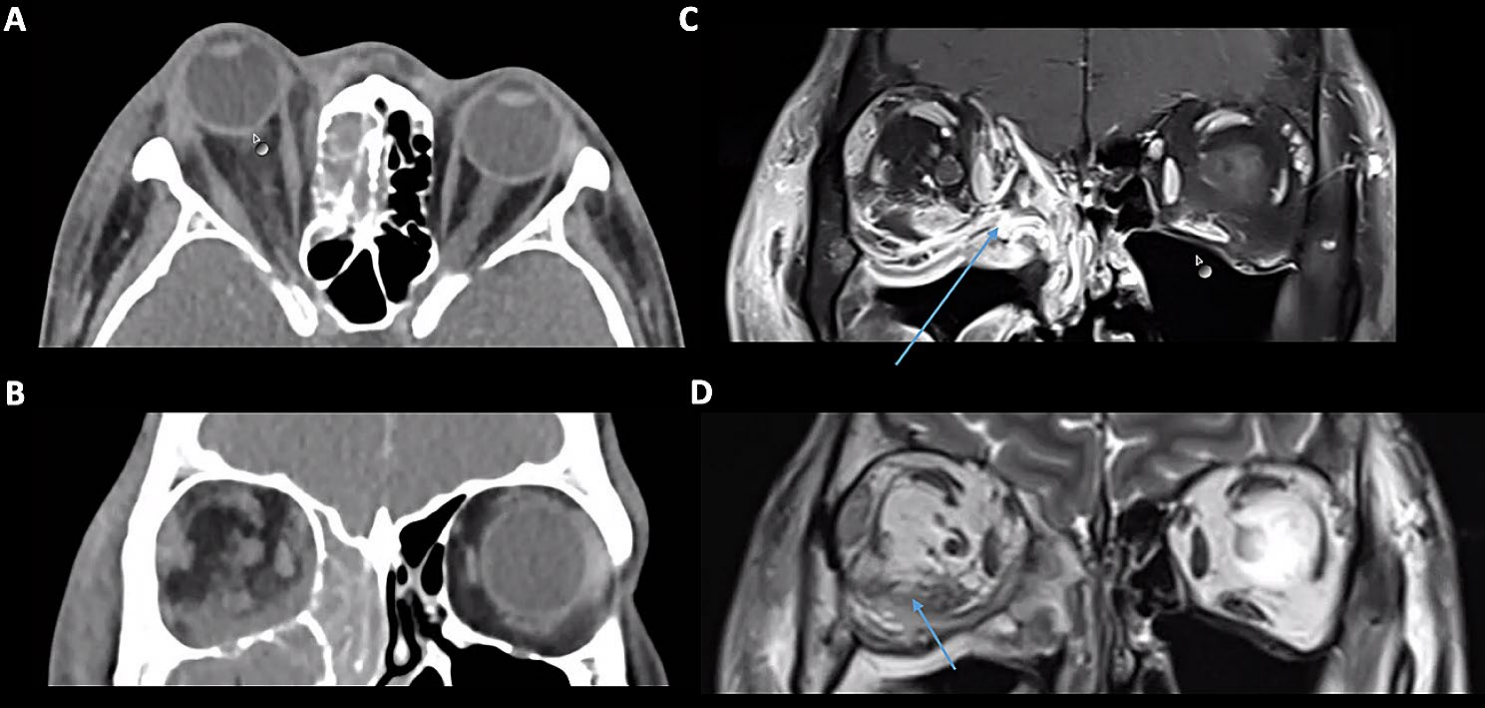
A-B: CT orbital scans. There is significant right sided proptosis with retrobulbar fat stranding and a subperiosteal abscess along the medial wall and orbital floor. Opacification of the right ethmoid and maxillary sinuses are also demonstrated. C: MRI orbital scan performed several days following admission. T1 fat-suppressed contrast-enhanced MRI demonstrating an enlarged and enhancing right inferior rectus and avid enhancement in the region of the subperiosteal abscess. D: Coronal T2 image shows hyperintensity of the right inferior rectus and adjacent subperiosteal abscess collection
